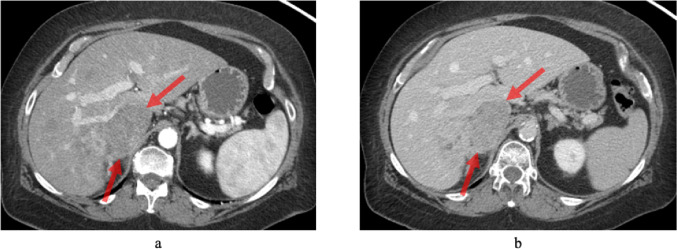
Intravascular Leiomyosarcoma. Axial contrast-enhanced CT images through the upper abdomen in arterial (a) and portal venous (b) phases demonstrate a large heterogeneously enhancing mass expanding and obliterating the lumen of the suprarenal IVC (red arrows). This is the imperceptible IVC sign, indicating an intravascular tumor
